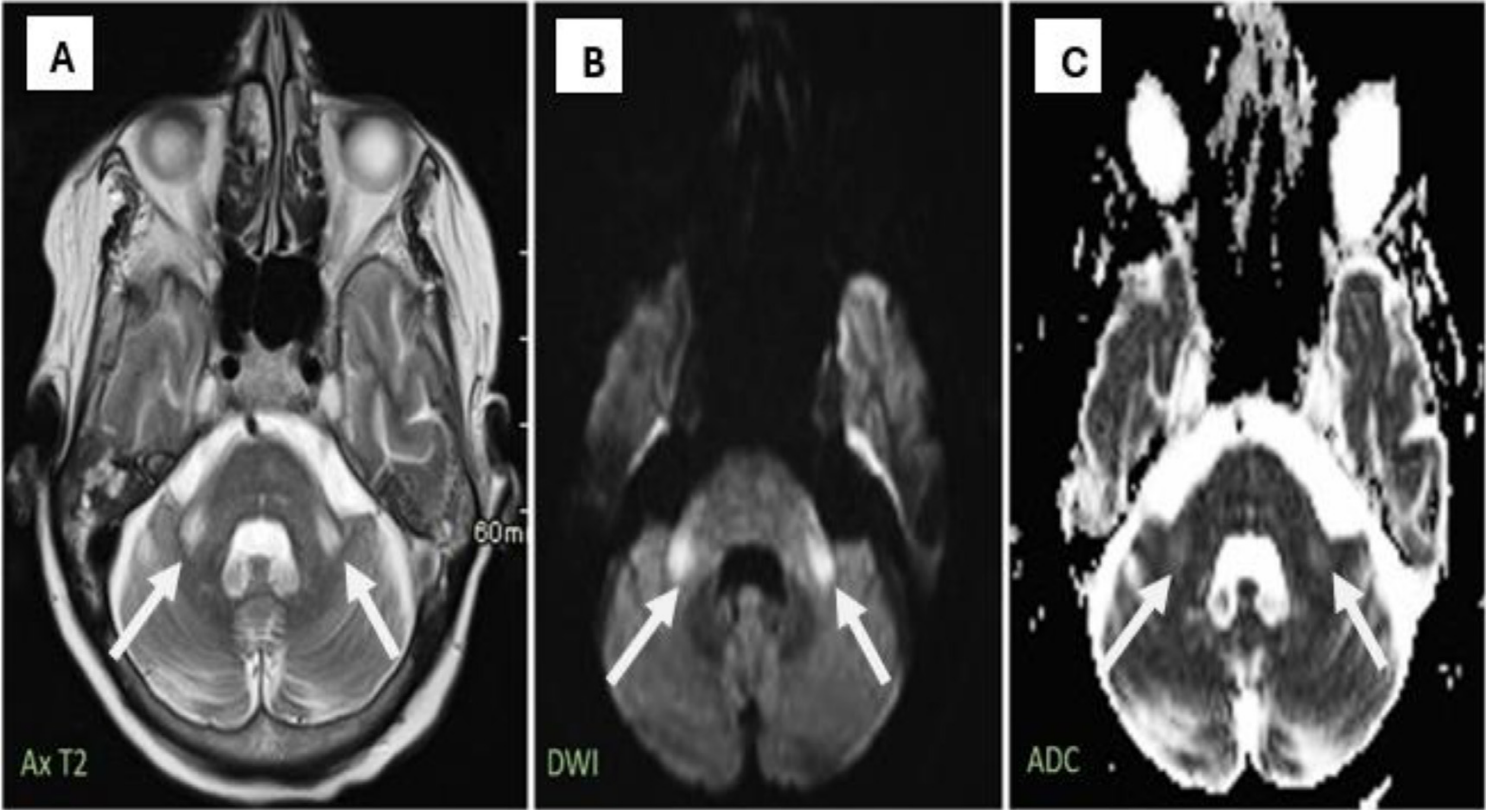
MRI findings of bilateral middle cerebellar peduncle Wallerian degeneration. (A) Axial T2-weight image (Ax T2) showing a symmetrical hyperintense signal in the bilateral middle cerebellar peduncles (white arrows). (B) DWI sequence and (C) ADC map revealing corresponding mildly increased signal intensity, typical of chronic Wallerian degeneration rather than acute infarction.
